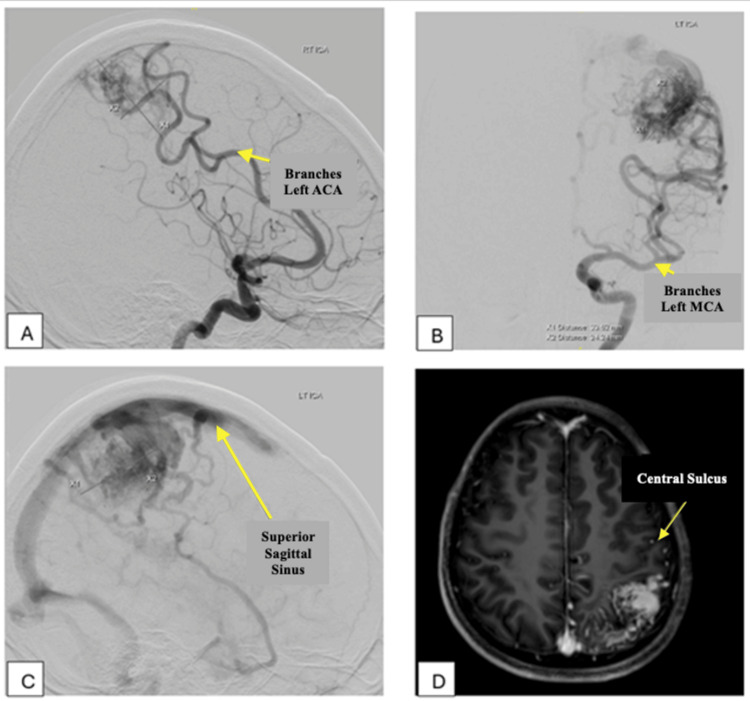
(A–C) DSA Brain and (D) MRI Brain show Spletzer Martin Grade 3 bAVM (D) evidenced by a nidus size of more than 3cm, eloquent region over postcentral gyrus and (C) cortical venous drainage into SSS. (A & B) Arterial supplies to the nidus via branches from the left ACA, MCA, and PCA. SSS: Superior sagittal sinus; ACA: anterior cerebral artery; PCA: posterior cerebral artery; MCA: middle cerebral artery
